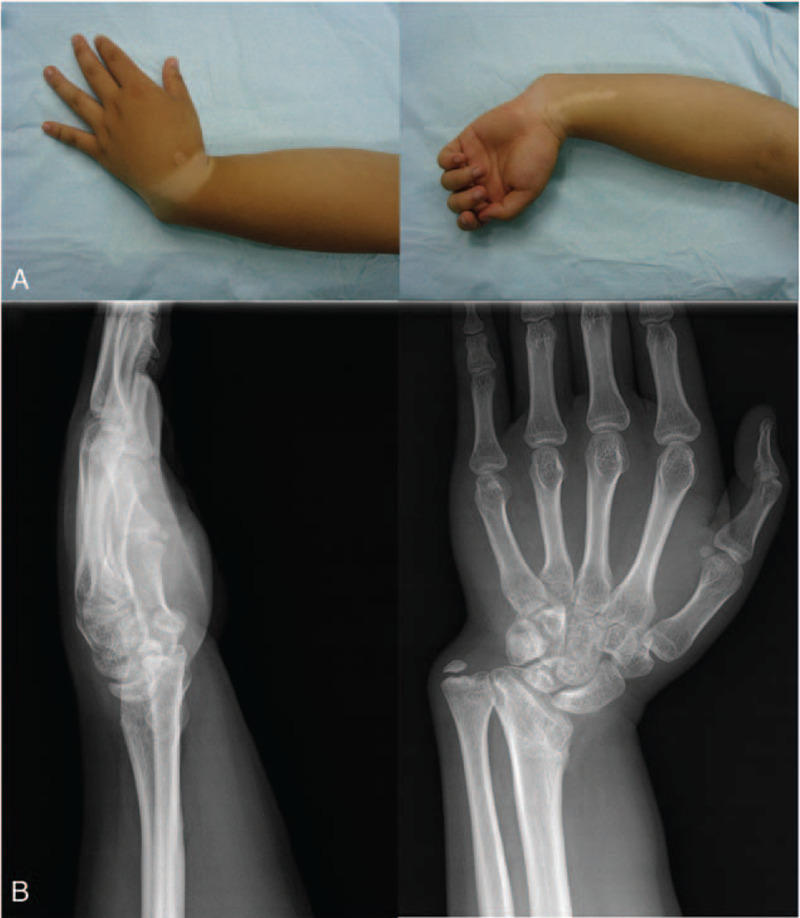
(A) Clinical pictures of serious cosmetic deformity of the left wrist. (B) The preoperative radiographs show deformity of the distal radius in both coronal and sagittal planes. Tilt of the radiocarpal articular surface and anatomical change of the carpal bones in the left wrist are shown in the images.
